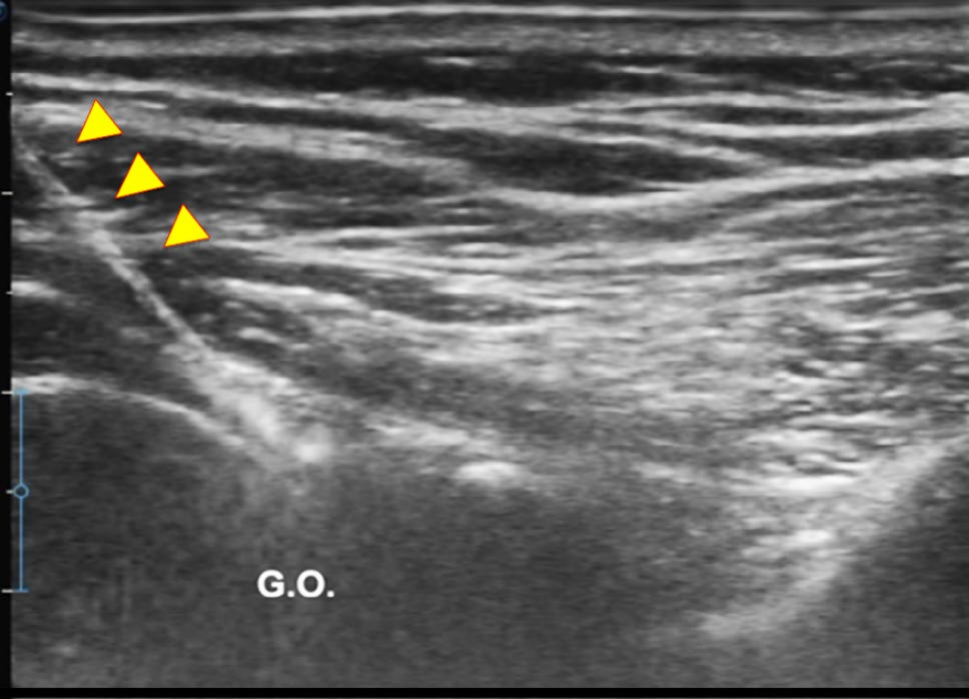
Ultrasound-guided infiltrative treatment: hydrodistention of the gleno-humeral joint through a posterior access to the shoulder joint
